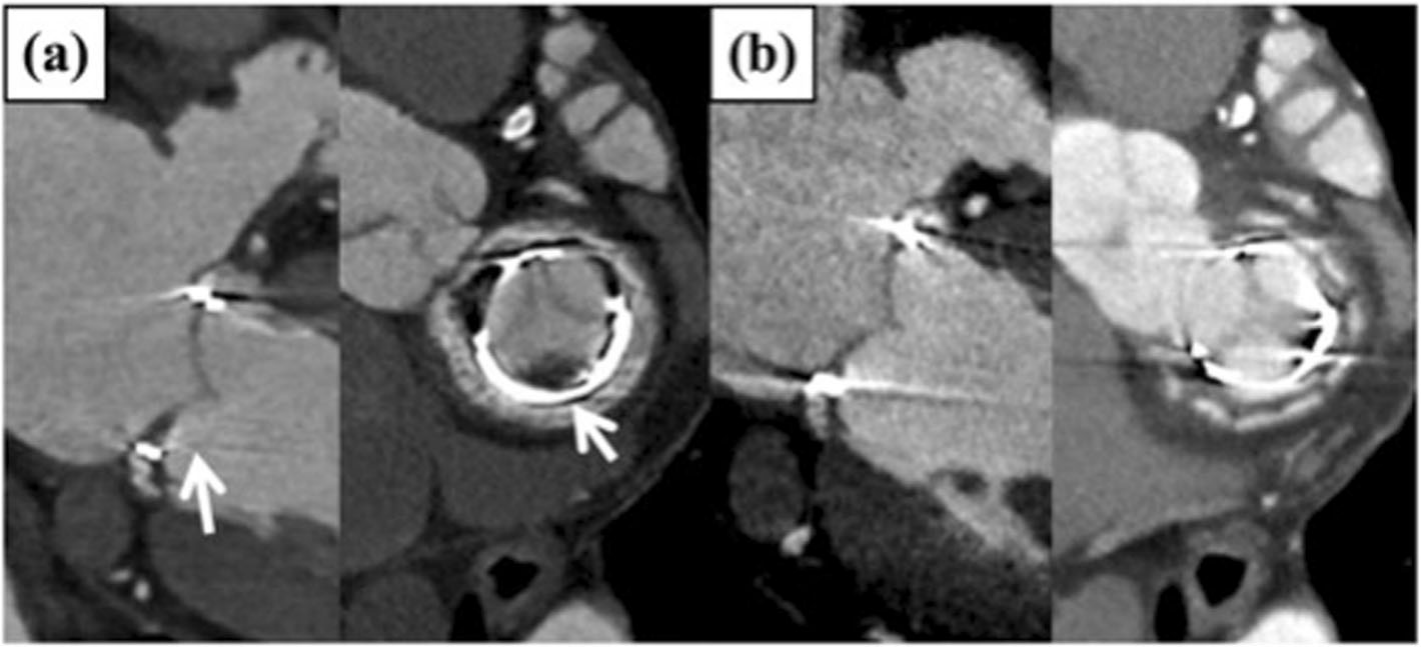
a initial CT in 65-year-old male. b Repeat CT scan was obtained 12 months after prasugrel and aspirin, and demonstrated complete resolution of the thrombus
